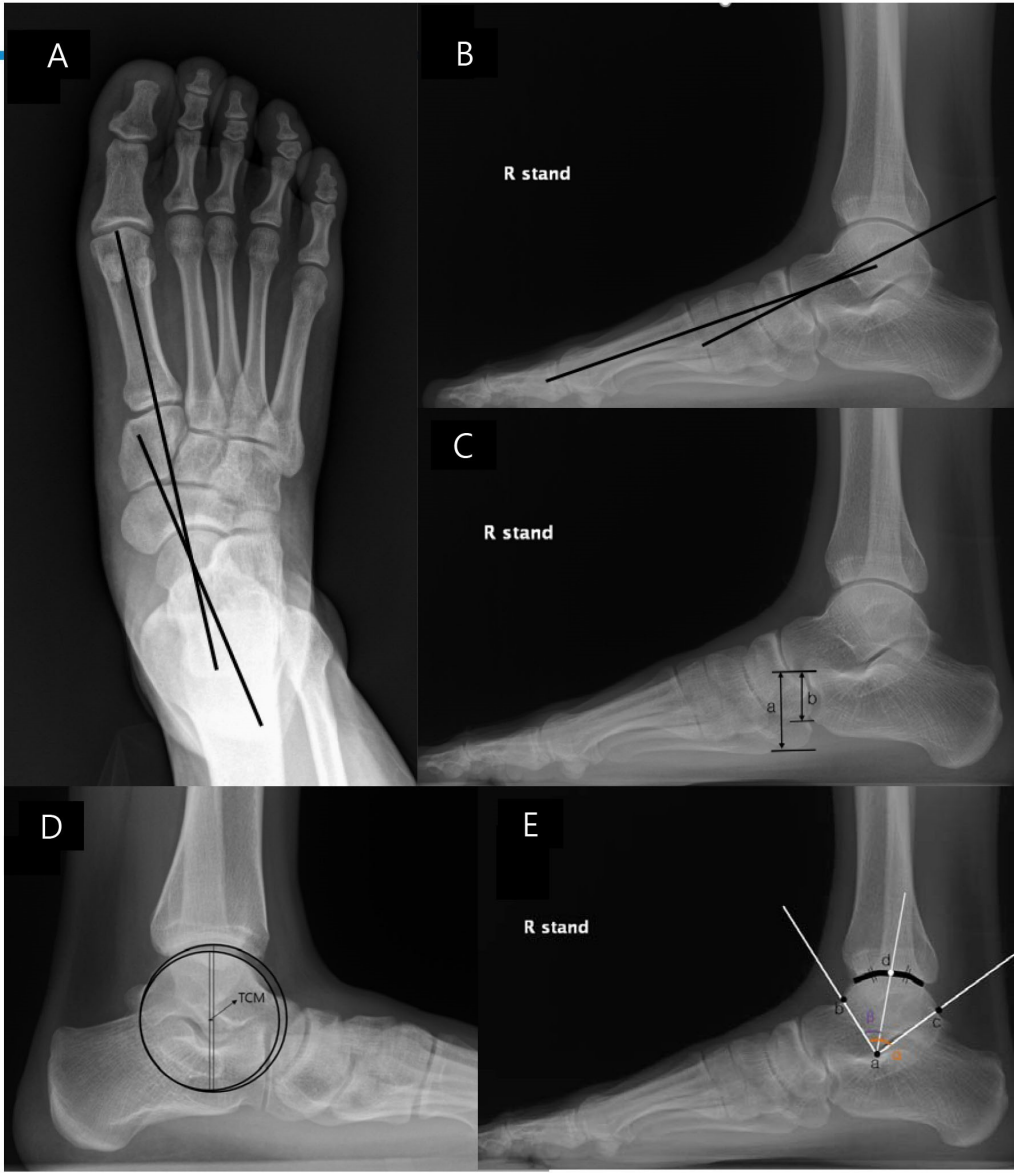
Radiographic measurements. A: AP talo-1MT is measured between the longitudinal axis of talus and that of the first metatarsal on the AP foot X ray. B: Lat talo-1MT is the angle between the longitudinal axis of the talus and that of the first metatarsal on the lateral foot X ray. C: NC overlap (b/a) is the vertical height of overlap between the navicular and cuboid (b) divided by that of the cuboid (a). D: ATCM is the anterior displacement of the center of a circle fitted to the tibial articular surface to that of another circle fitted to the talar articular surface. E: Position of the articulating surface is the angular position of the bisecting point of the contact surface between the tibia and talus within the total arch of the talar articulating surface (β/α; a: tip of the talar lateral process, b: anterior tip of the talar articular surface, c: posterior tip of the talar articular surface, d: bisecting point of the contact surface between the tibia and talus).Two orthopedic surgeons, with 6 and 3 years of orthopedic experience, participated in the reliability testing. After a consensus-building session for radiographic measurements, each surgeon performed radiographic measurements without any clinical information for a predetermined number of radiographic images that were presented in random order by a research assistant who was not involved in this study. One of the surgeons measured the radiographic parameters for all patients pre-and postoperatively, following interobserver reliability testing.
